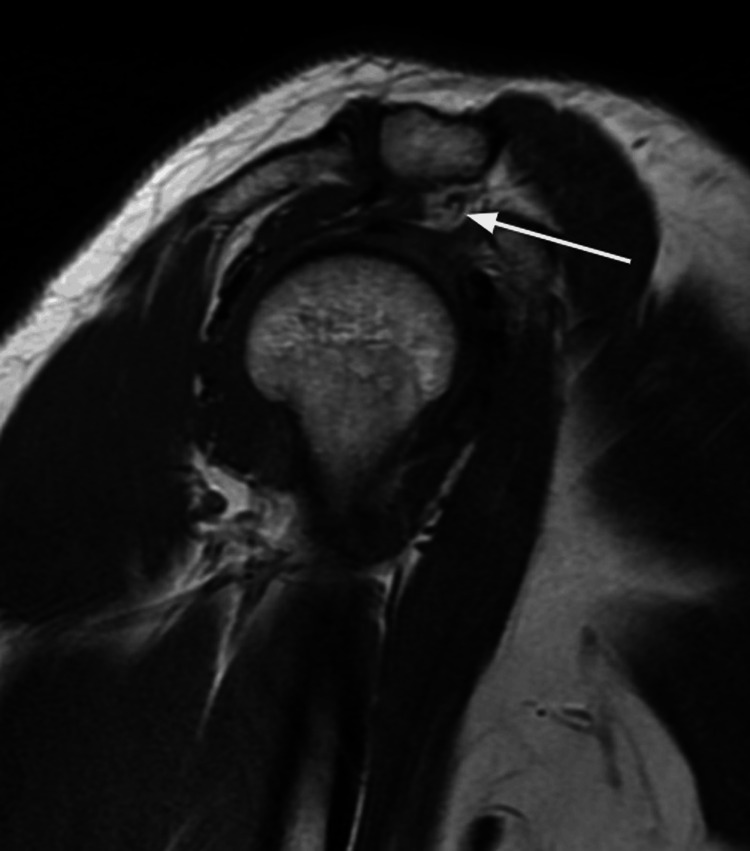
Sagittal T1-weighted MRI. The white arrow indicates obliteration of the rotator interval.
